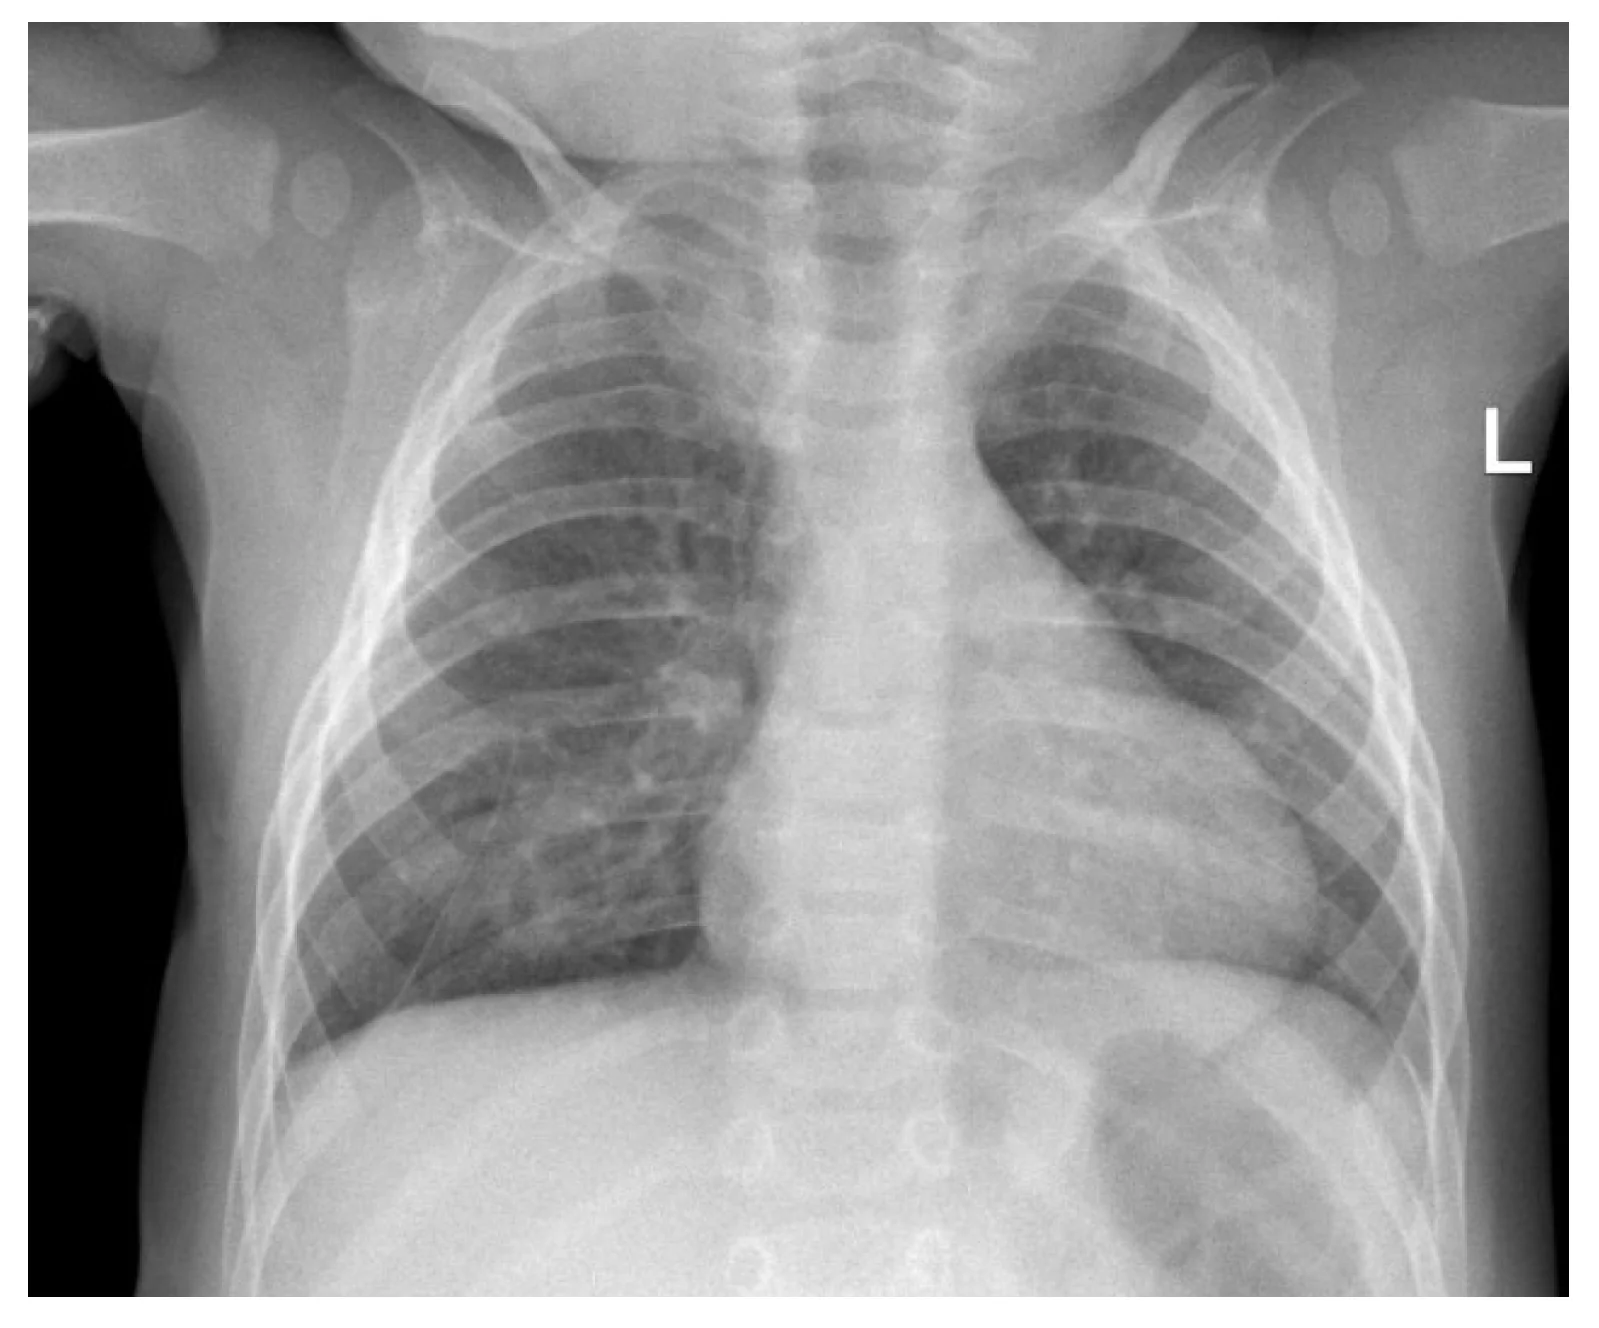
Posteroanterior chest radiograph of an 11-month-old male with acute right-sided pneumonia.
